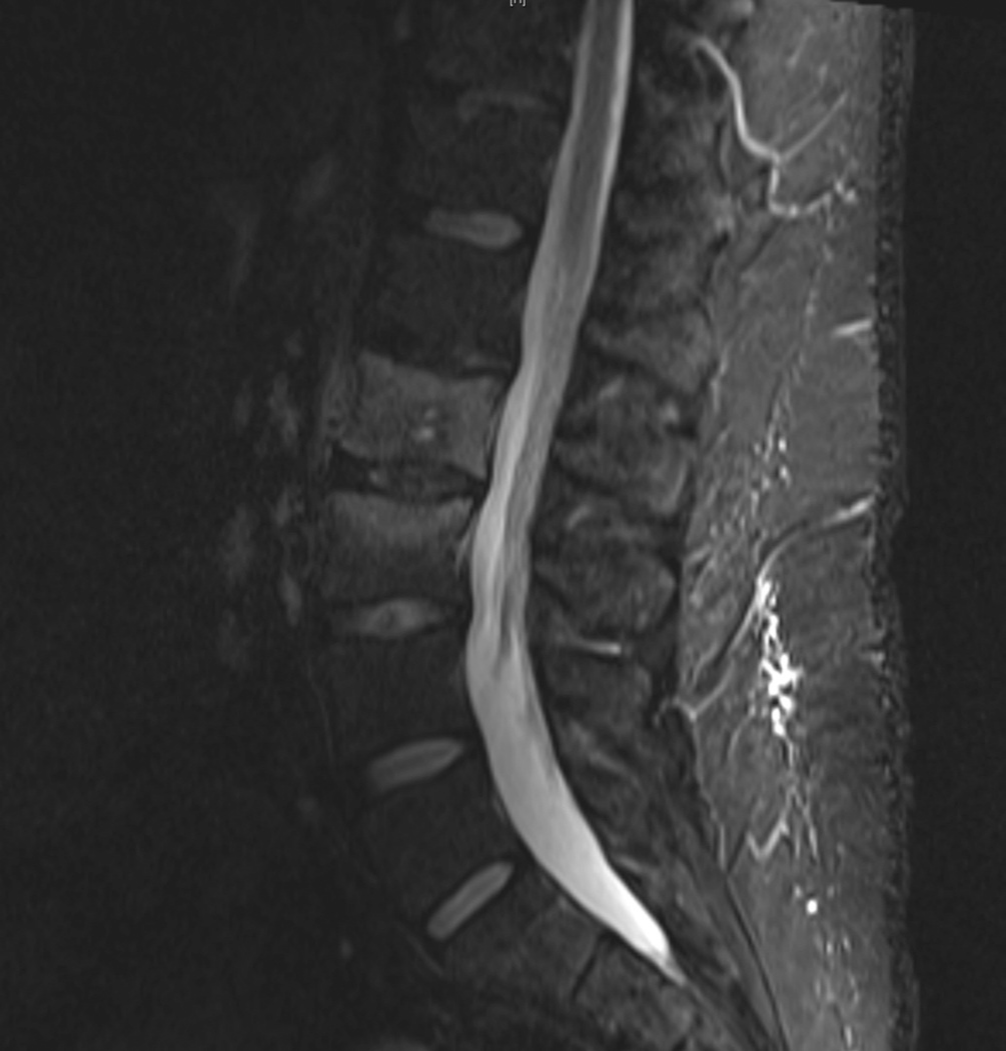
Follow-up MRI demonstrating increased signal change within the L2 vertebral body.
